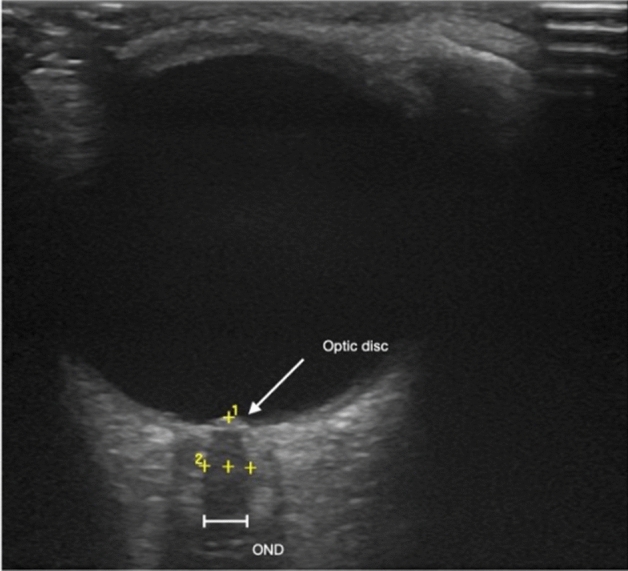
Example measurement of optic nerve diameter with transorbital ultrasonography. Optic nerve diameters were measured at a 3 mm distance behind the optic disc in a longitudinal view of the nerve.
